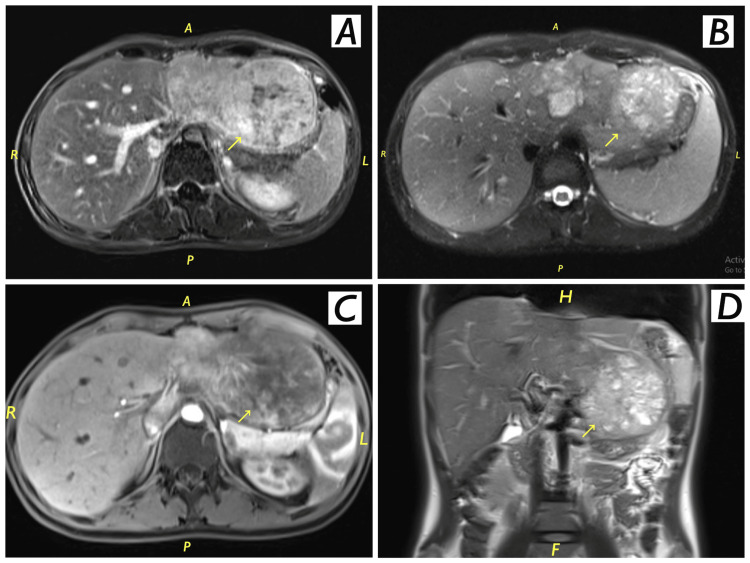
Abdominal MRI findings confirm hepatocellular carcinoma Abdominal MRI showing non-cirrhotic liver with a smooth outline; there is a large, well-defined exophytic mass with lobulated margins in the left lobe of the liver, measuring about 11.4 x 7.2 x 8.8 cm in maximum transverse, and craniocaudal view as shown in 2A, 2B, 2C and 2D.
